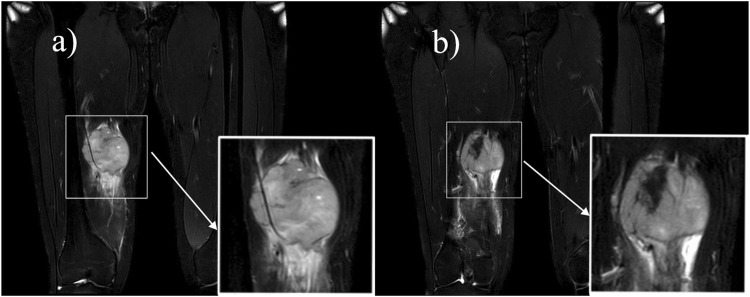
MRI: A soft-tissue mass of the medial muscle of the right mid-thigh, which was lobulated and showed a “Triple-sign” of mixed high and low signals on T2-weighted images. (A) Speckled high signal is seen within the lesion, suggesting a localized cystic lesion, and the lesion encircles the superficial femoral artery. (B) Fibrous components and old hemorrhages in the lesion show low signal.
